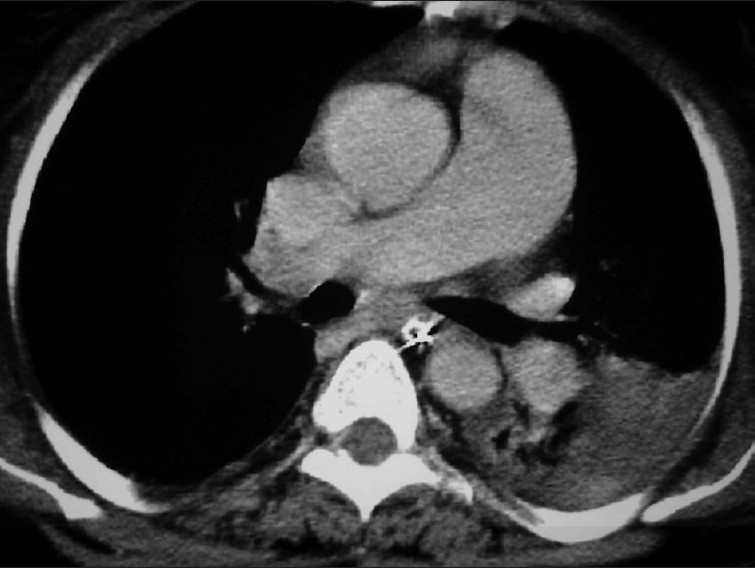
Contrast enhanced CT scan thorax at the level of carina showing consolidation and effusion on left upper lobe
